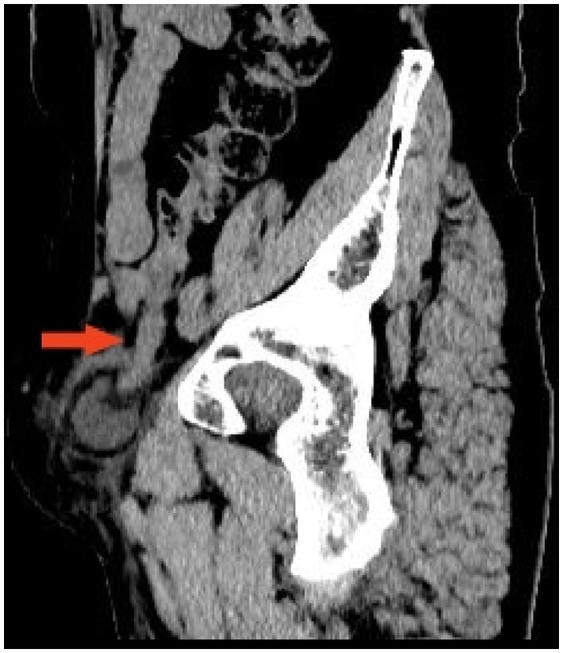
Abdominal CT coronal image of the appendix bursting into the right groin.
